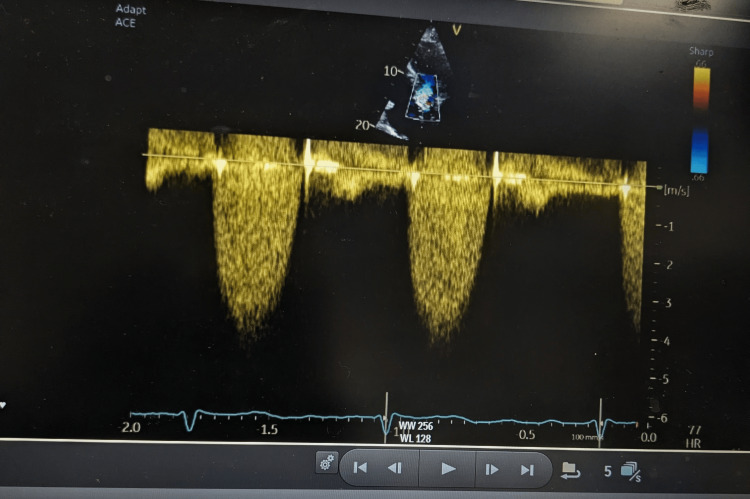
Continuous wave Doppler at the aortic area showing a peak velocity of 4.0 m/s
